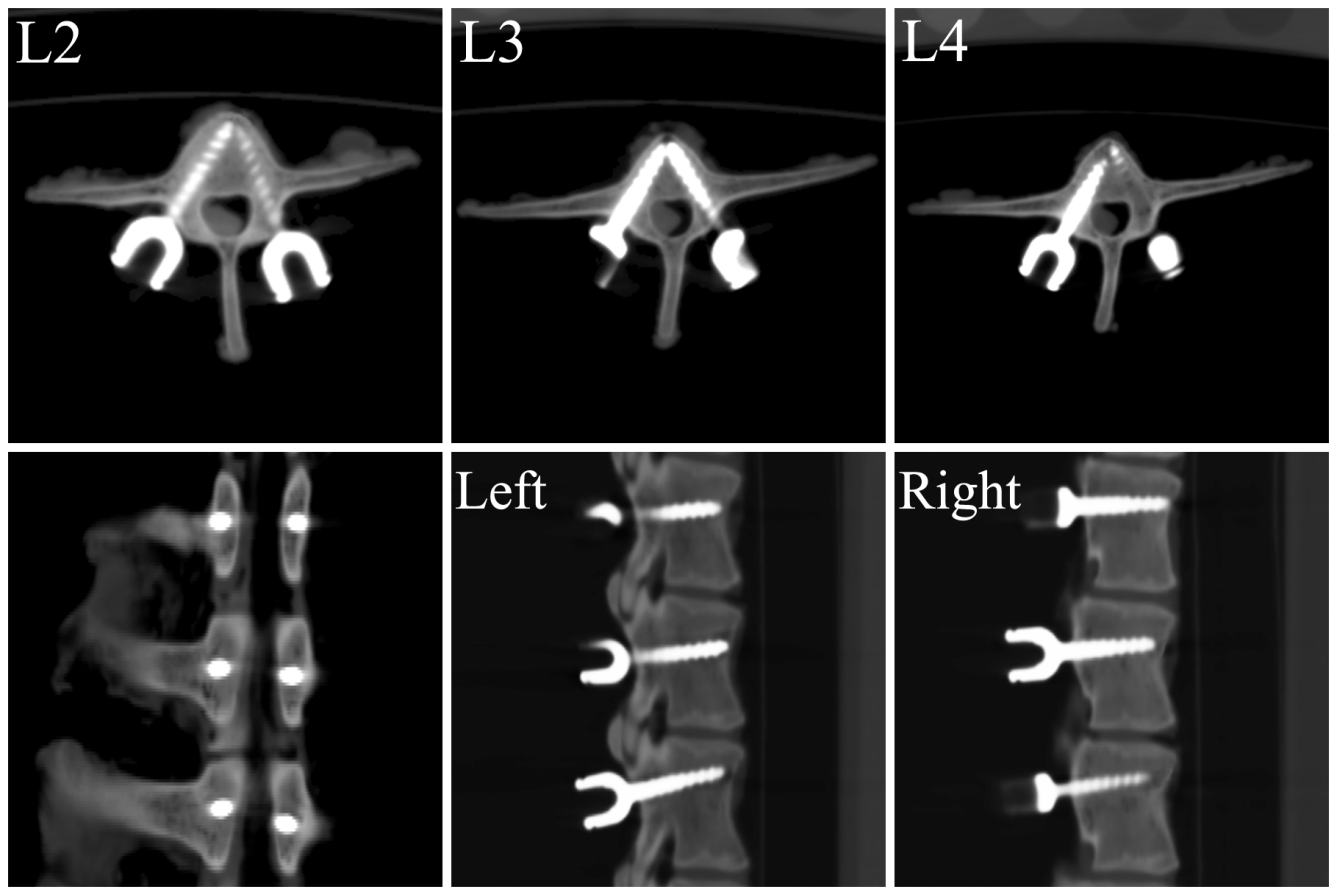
Screw position accuracy measurements as determined on postoperative CT scans. A, Planned merged over actual placement. B, Comparison between the panned and inserted screw at the entry point and angles.
